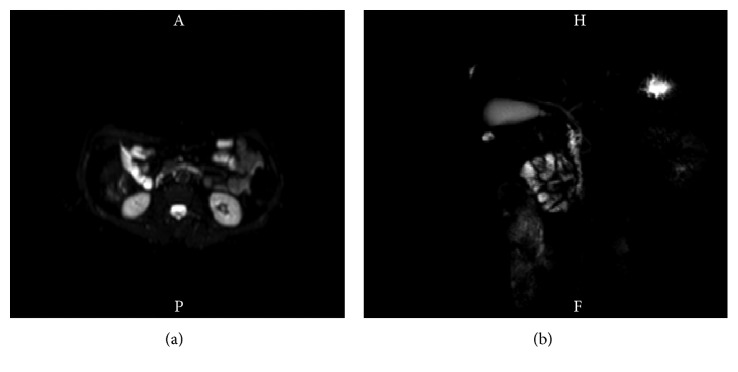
Normal representative magnetic resonance imaging of the (a) abdomen and (b) bladder and bile duct.
